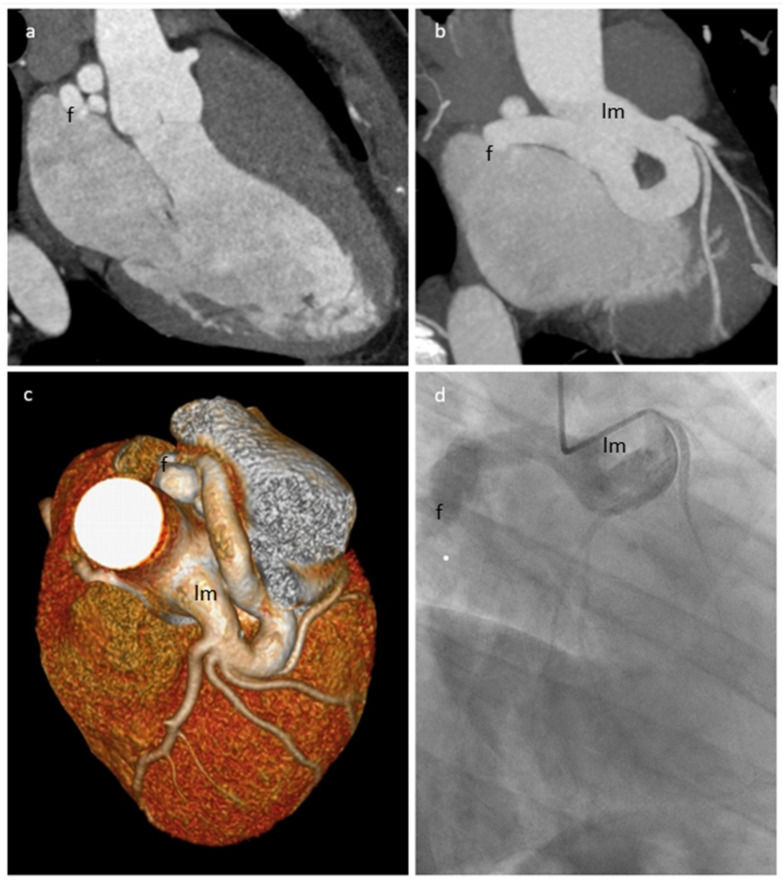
A 28 year-old male patient with a left main (lm) coronary artery to left atrium fistula (f) in computed tomography three-chamber view (a), curved planar reconstruction (b), volume-rendering (c), and coronary angiography (d).
